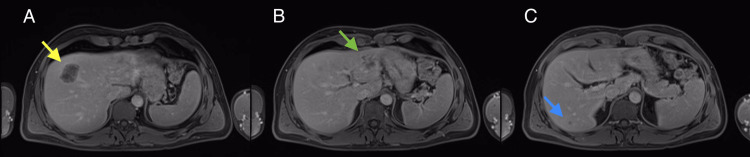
MRI axial plane A: Lesion at the interface of segments 8/4a. B: Lesion in segment 2. C: Lesion in segment 6. MRI: magnetic resonance imaging
